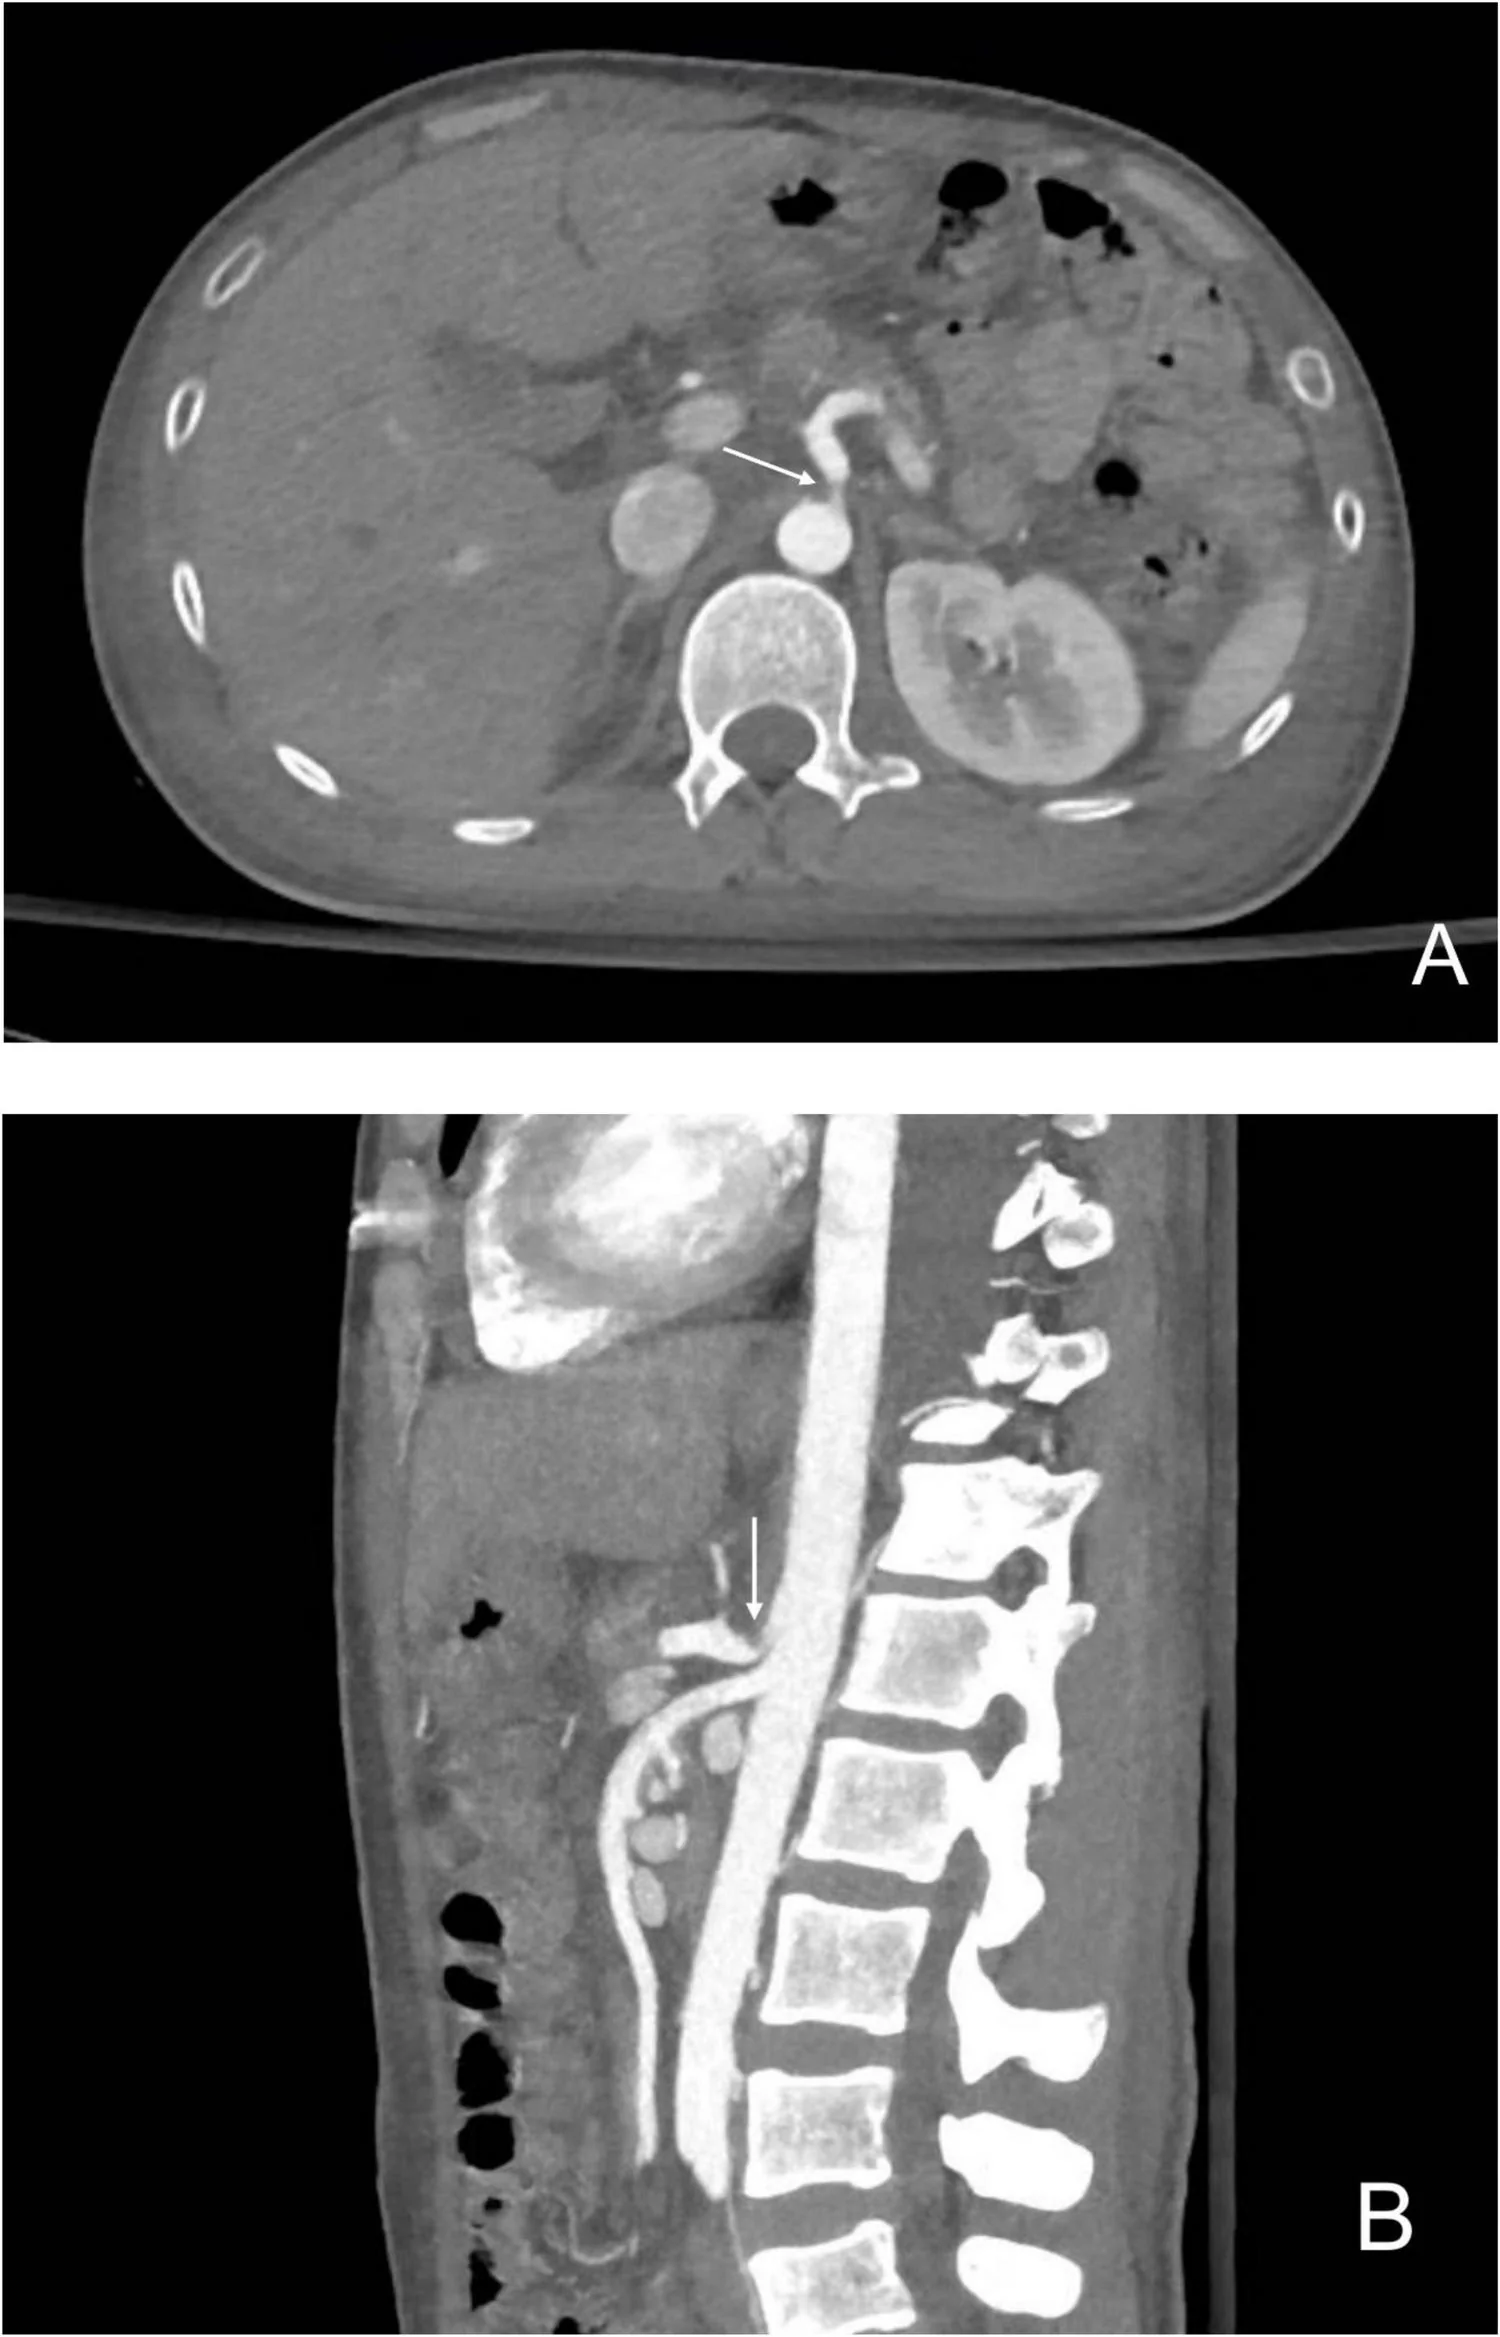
(A, B) Results of abdominal CT angiography. Repeated abdominal CT angiography demonstrated high-grade stenosis of the proximal celiac trunk, obvious fish-hook configuration and post-stenotic dilatation, although the median arcuate ligament could not be adequately assessed because of limited image quality.
